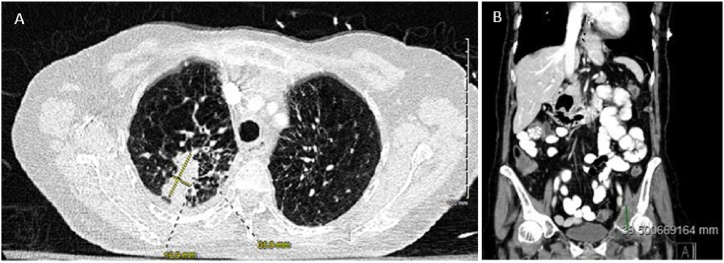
A-B: Computed tomography (CT) angiogram of the chest reveals two masses in the right lung apex measuring 2.3 × 2.1 cm and 3.6 × 1.7 cm (A), and a CT of the abdomen and pelvis demonstrates a destructive lytic lesion of the left acetabulum measuring 3.9 × 3.4 cm (B).
